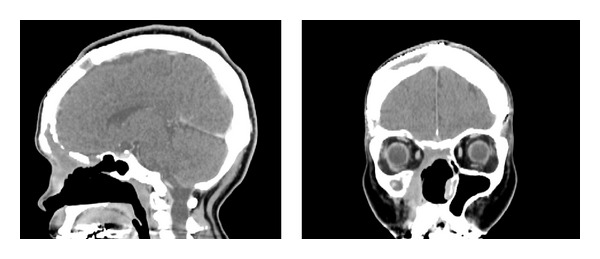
Postoperative computed tomography (contrast-enhanced) shows no recurrence that is seen in both nasal cavities and the intracranial space.
